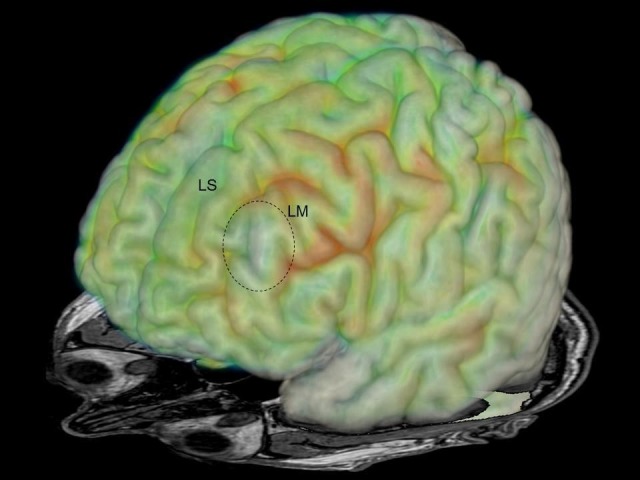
Image fusion of MRI and FDG-PET Image fusion of MRI and FDG-PET demonstrating hypometabolisms in the left medial frontal gyrus (dotted circle). LS: left superior frontal gyrus; LM: left middle frontal gyrus; MRI: magnetic resonance imaging; FDG-PET: fluorodeoxyglucose positron emission tomography
